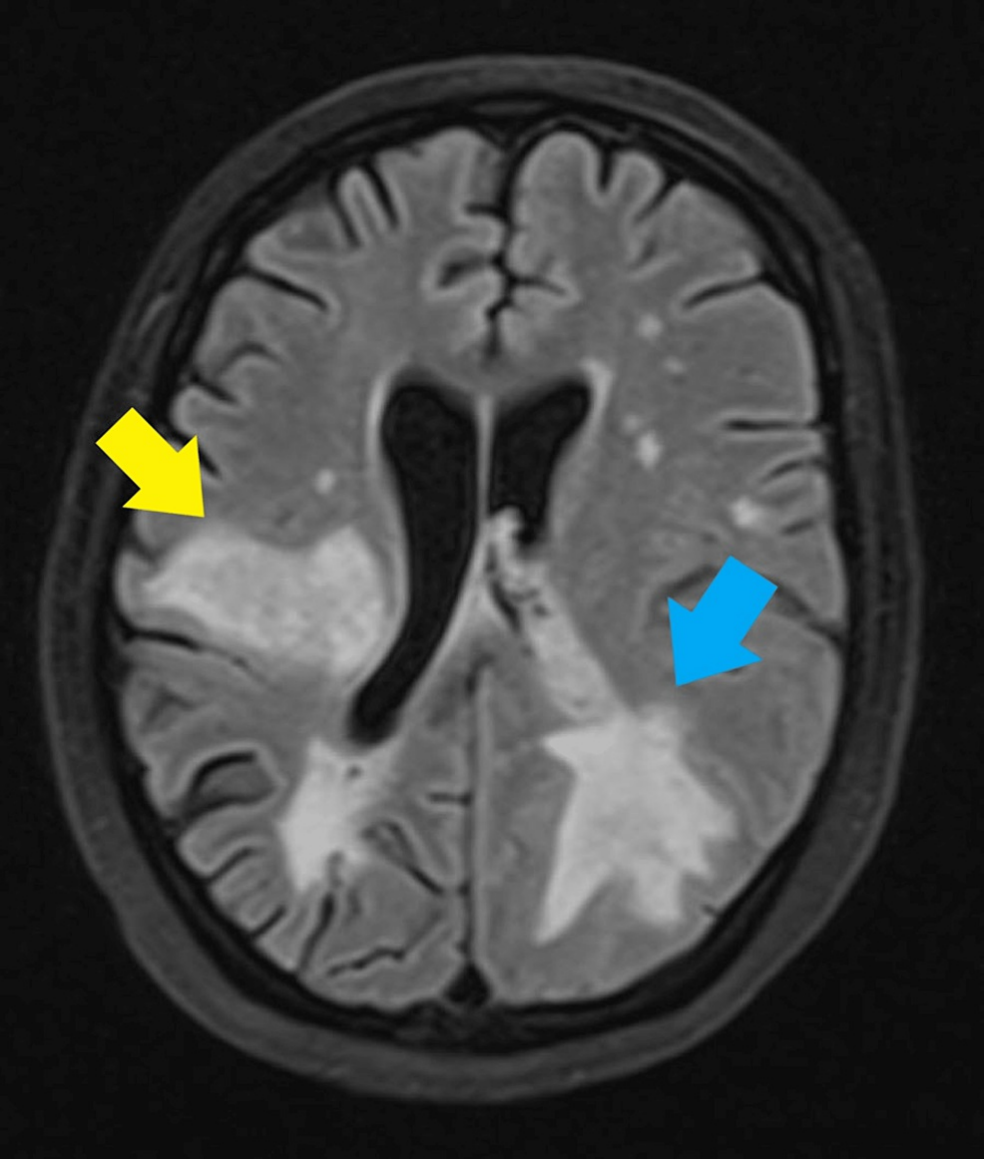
MRI brain axial view The yellow arrow shows the location of the abscess and the blue arrow shows the location of intracerebral hemorrhage with intraventricular hemorrhage
